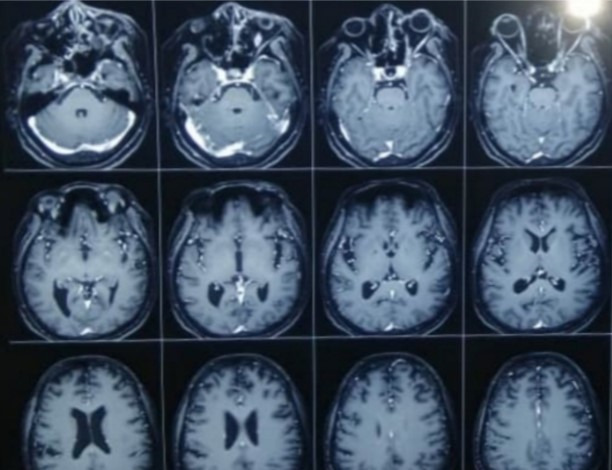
Contrast enhanced MRI of brain, orbits and PNS showing no intracranial or intraorbital involvement
